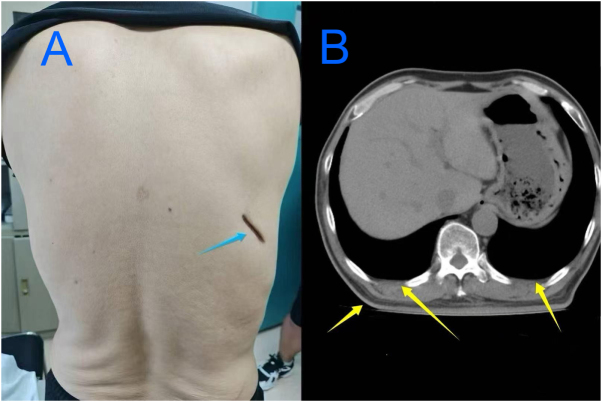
Follow-up findings 2 months after incision and drainage of the chest wall abscess. (A) Photograph of the right posterior chest wall showing the healed incision site (blue arrow). (B) Chest computed tomography scan showing resolution of the chest wall abscess and a reduction in the size of the bilateral pleural effusions (yellow arrows).
